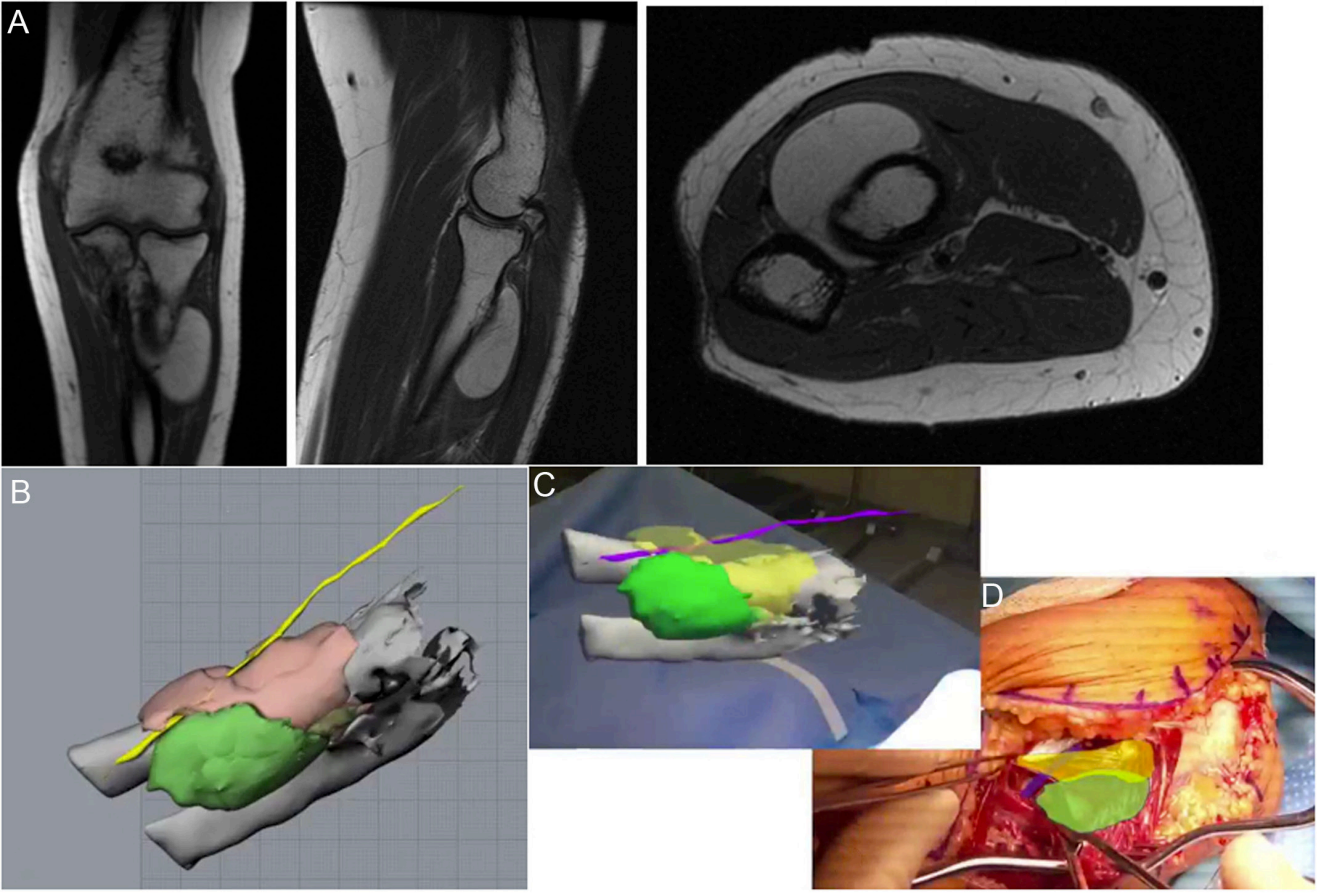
(A) Preoperative MRI demonstrating a well-circumscribed forearm mass within the supinator muscle. (B) MRI was used for 3D preoperative modeling to identify the course of PIN relative to the mass. (C) Intraoperative hologram demonstrating MR assistance. (D) The 3D model is positioned within the surgical field, highlighting the mass (green), supinator (yellow), and PIN (purple).
